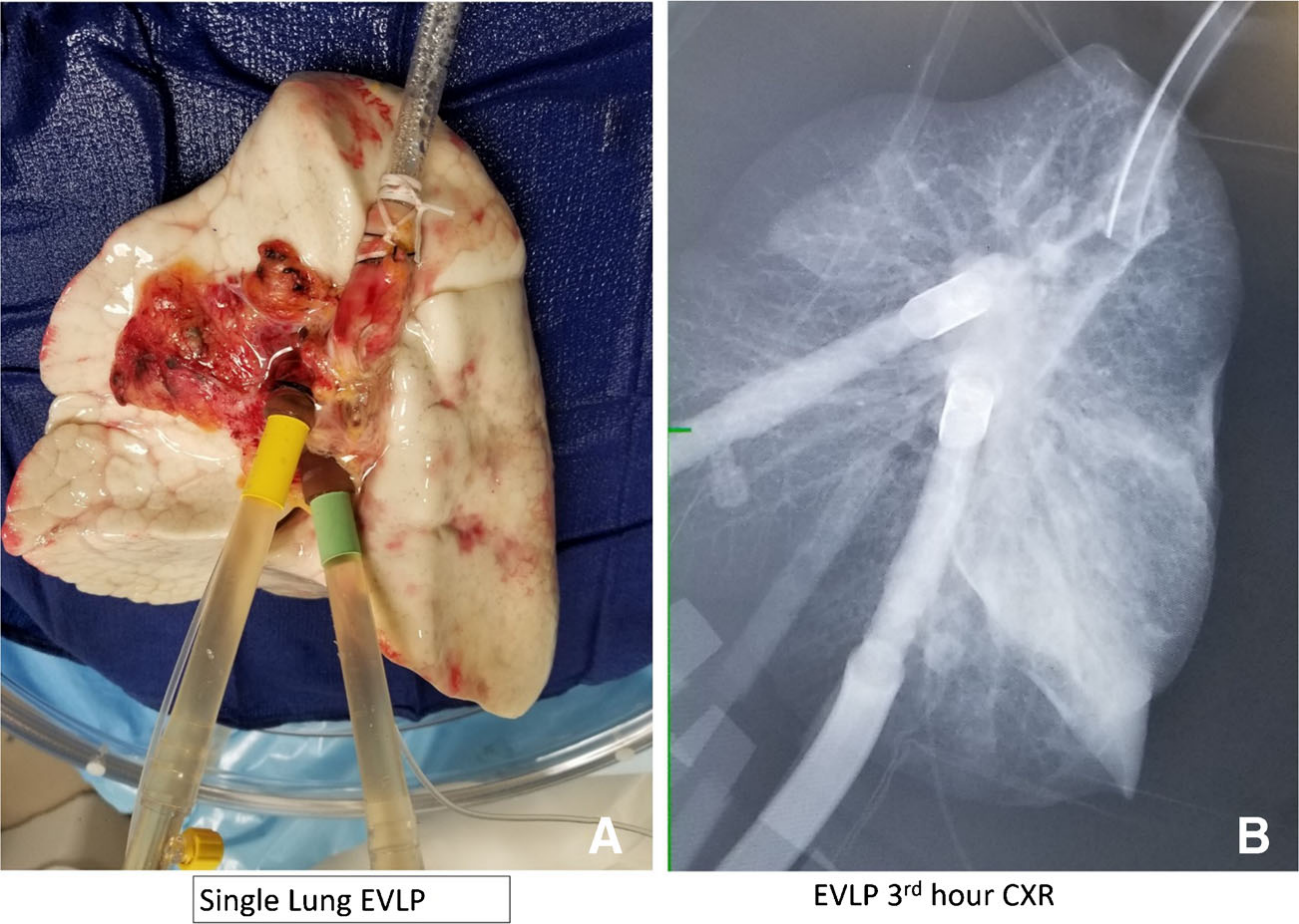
A Single lung EVLP run. B CXR after 3 h showing dense consolidation in the lower lobe (EVLP, ex vivo lung perfusion; CXR, chest X-ray)
